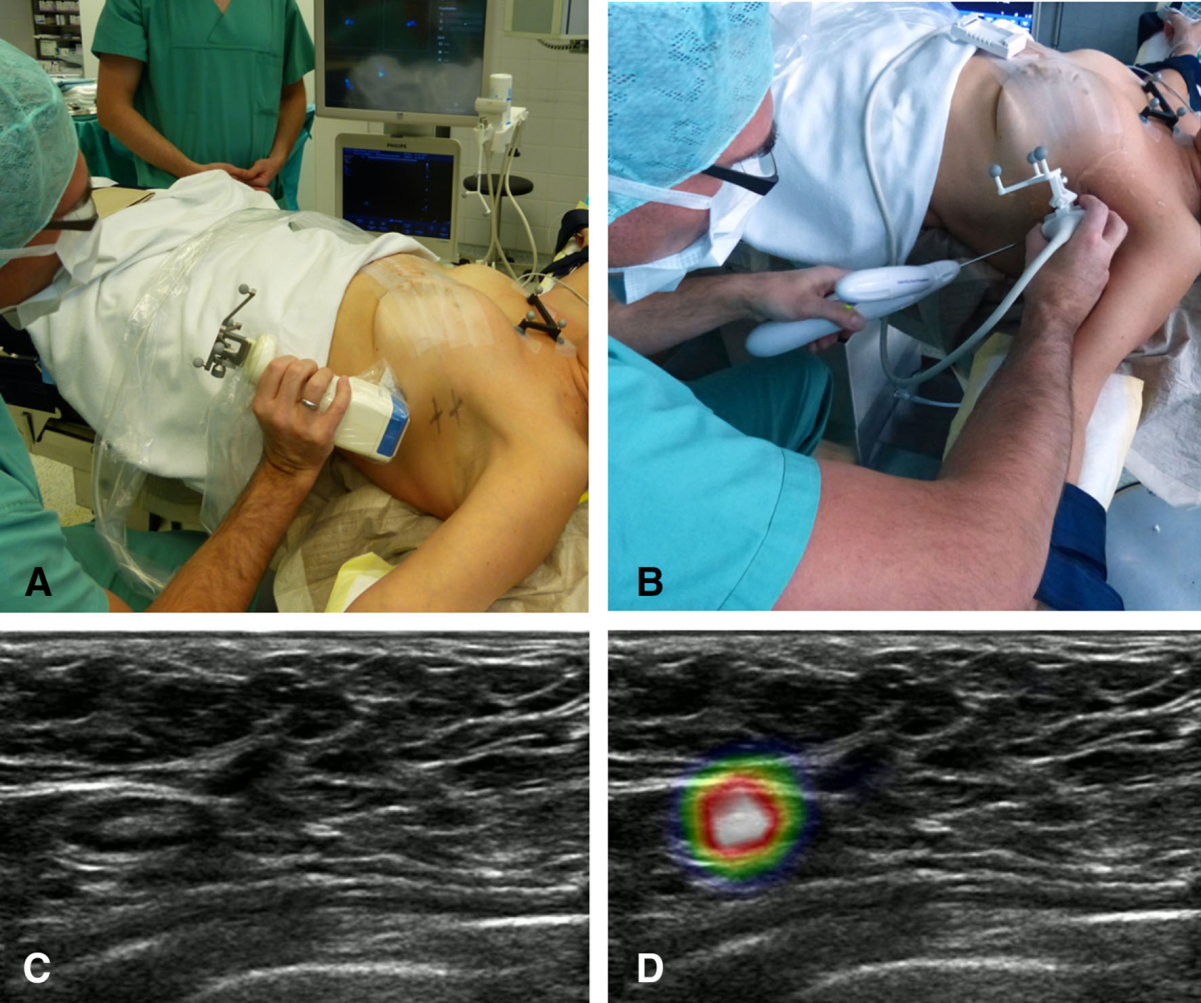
Freehand SPECT–ultrasound in action during sentinel lymph node aspiration biopsy. a Freehand SPECT acquisition using a handheld gamma camera as nuclear detector, in a breast cancer patient. b Placement of the needle for aspiration biopsy based on freehand SPECT–US image. c B-mode image of axilla of patient showing at least one lymph node. d Freehand SPECT–US combination highlights the radioactive SLN by making it more prominent (Figure reproduced with permission from [19]—Wendler. Intraoperative 3D nuclear imaging and its hybrid extensions)
